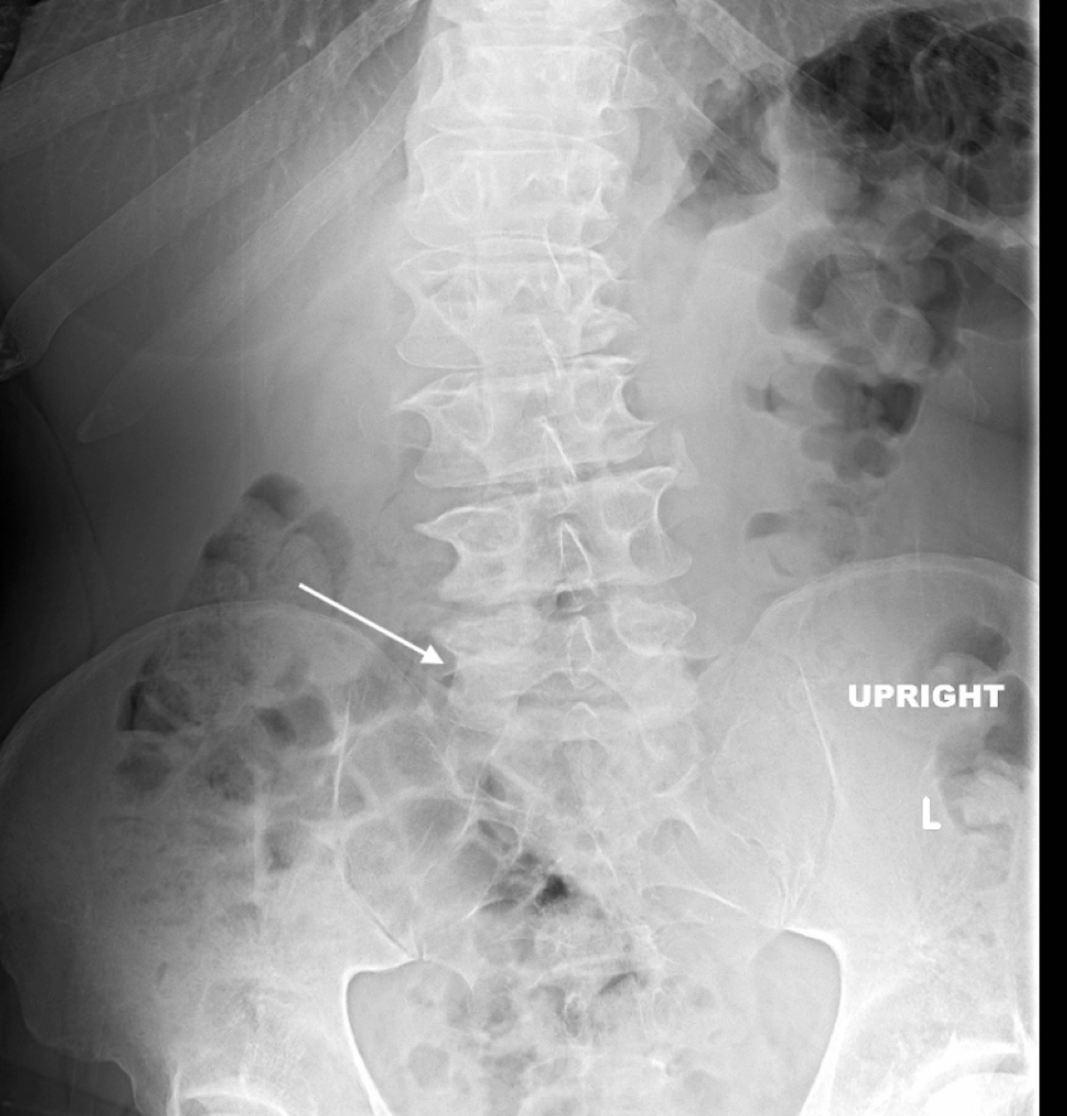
X-ray of the lumbar spine showing only degenerative changes (white arrow). The absence of evidence of the ongoing spinal infection is notable.
